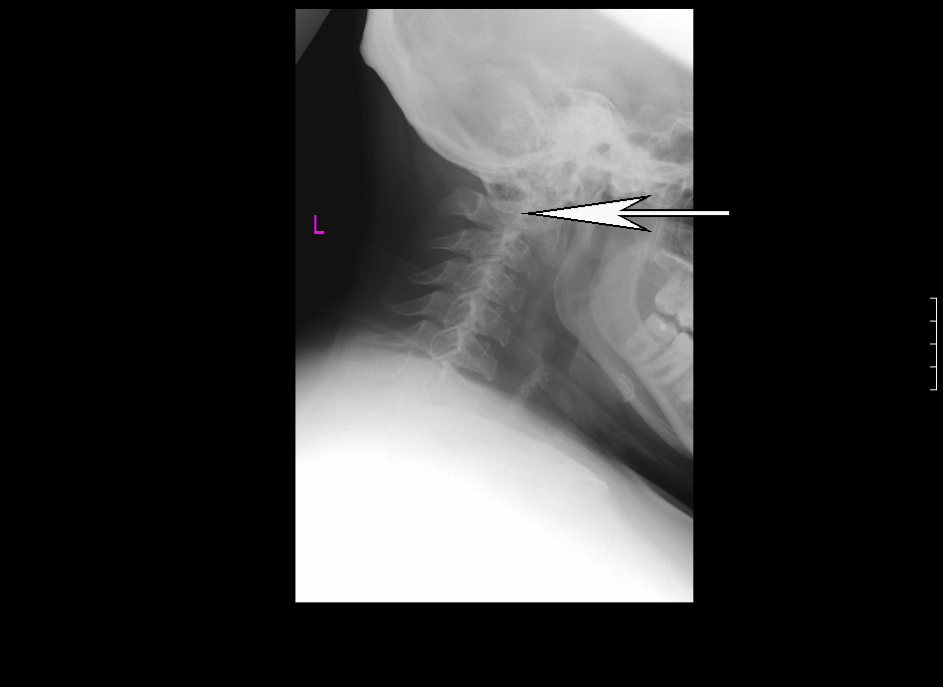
Lateral cervical spine x-ray demonstrating loss of normal cervical lordosis, anterior beaking of the cervical bodies and hypoplasia of odontoid peg. The atlanto axial space on flexion is 3 mm, the upper limit of normal.
